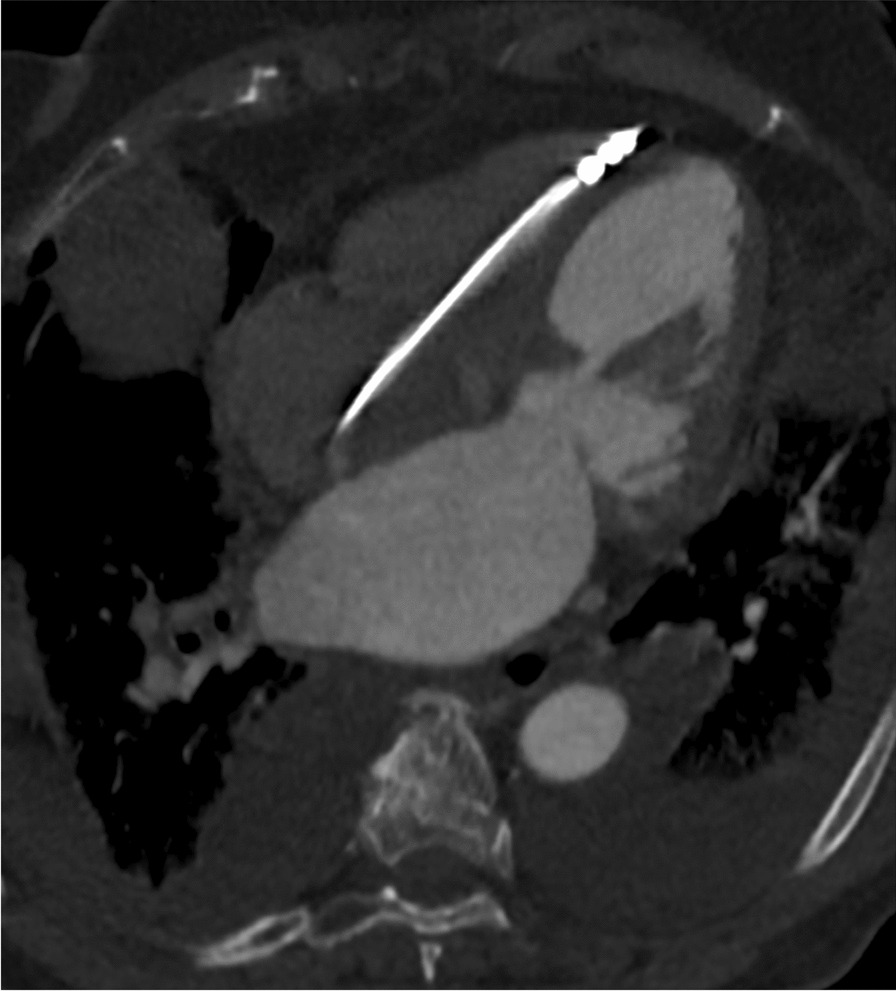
Noncontrast computed tomography scan of the chest confirmed the position of the lead of the pacemaker in the right ventricle
